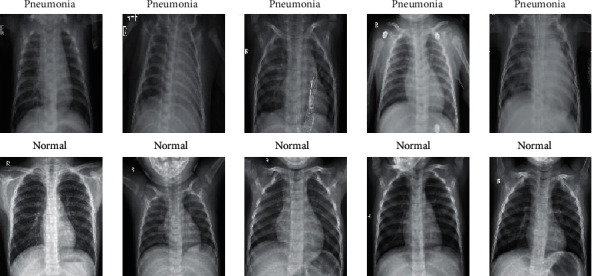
Chest X-ray images (pneumonia/normal).
